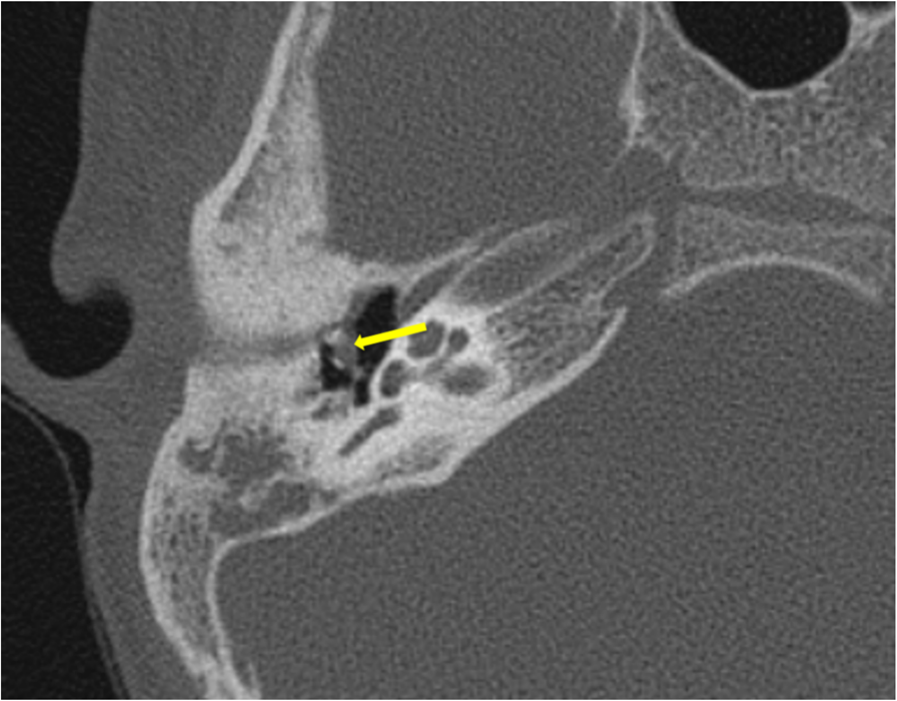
Axial CT image showing non-dependent soft tissue medial to ossicles in a patient with pars tensa cholesteatoma (arrow)
